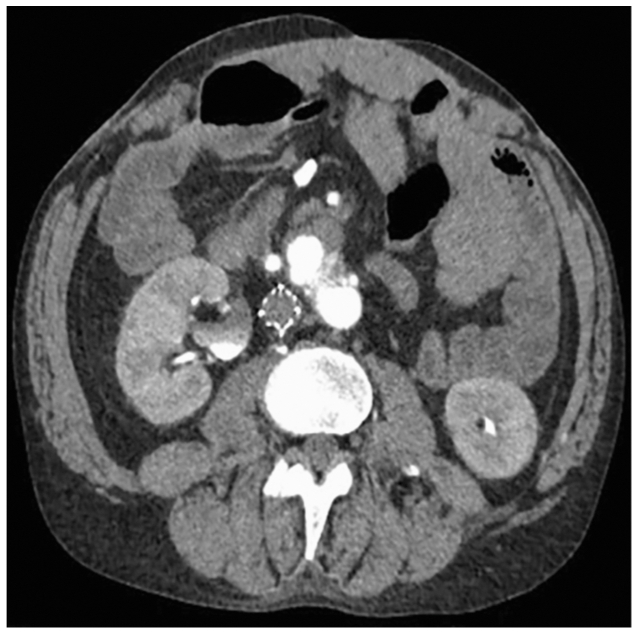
Computed tomography (CT) angiogram of the abdomen and pelvis before intervention.
